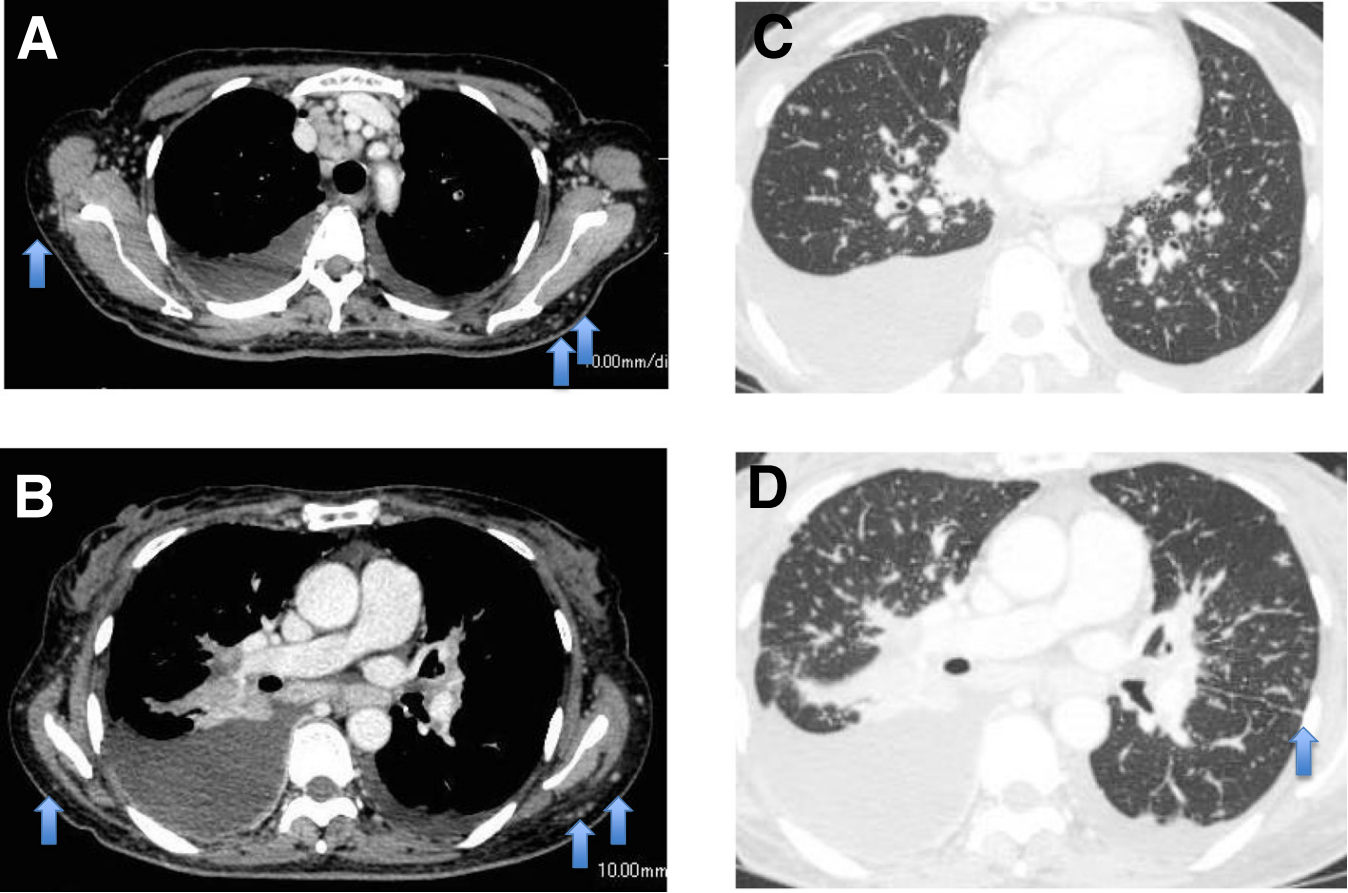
Chest computed tomography findings on admission. a and b: Multiple mediastinal and bilateral hilar lymphadenopathy, bilateral pleural effusion, and multiple small subcutaneous nodules indicated by *arrows*. c and d: Bilateral multiple small nodular shadows in the lungs and multiple small nodules along the interlobar pleura (*arrow*)
